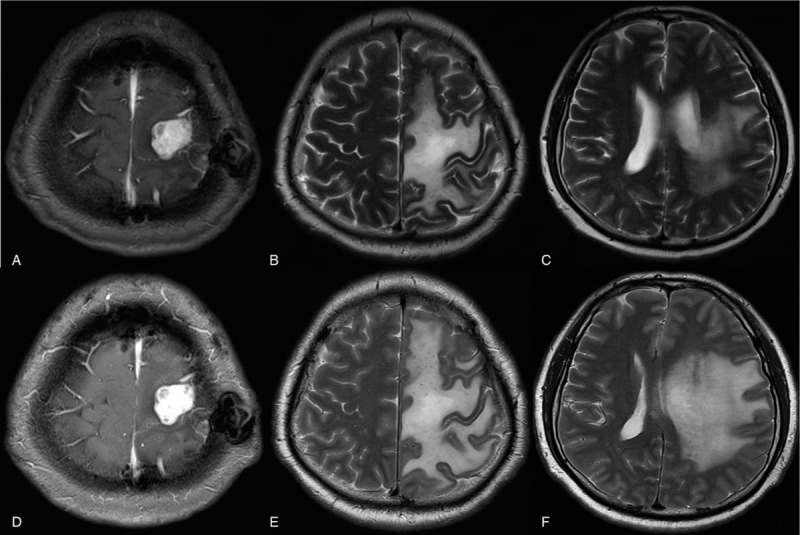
There was a slight decrease in T2 high signal intensity on MRI after 8th cycle of bevacizumab (A–C). However, peritumoral edema was worsened again on MRI despite additional 4 cycles of bevacizumab (D–F).
